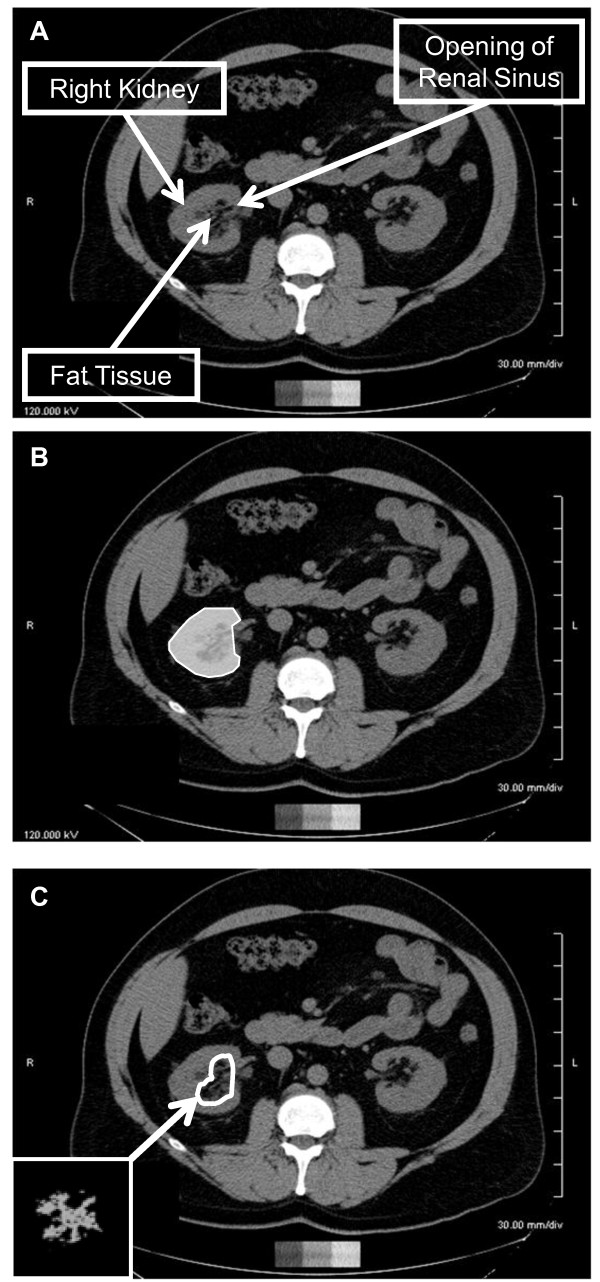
Abdominal computed tomography scans demonstrating the renal sinus fat measurement technique. A: Example of single abdominal computed tomography slice selected for renal sinus fat measurement. B: One measurement was taken in the right kidney. The reader manually traced within the border of the kidney. C: Visualization of renal sinus fat in slice measured in B using the Aquarius Workstation body fat analysis template (TeraRecon, Inc, San Mateo, CA, USA). The renal sinus is traced in this figure, highlighting the visualization of fat tissue observed in this region of interest.
